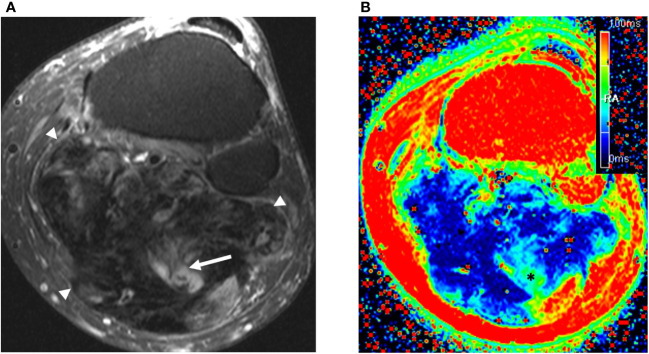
57 year old man with large calf mass. (A) Axial fat suppressed T2-weighted weighted MRI demonstrates a large mostly collagenized desmoid tumor in the calf (arrowheads). The tumor:muscle signal ratio was 0.34. Note small areas of internal T2 hyperintensity suggestive of more cellular components (arrow). (B) T2 mapping reveals low signal throughout the tumor, with areas of higher signal (*) corresponding with the T2 hyperintense components seen in (A) The T2 relaxation time of the tumor was measured by readers 1 and 2 as 33.6 ms and 29.3 ms, respectively.
